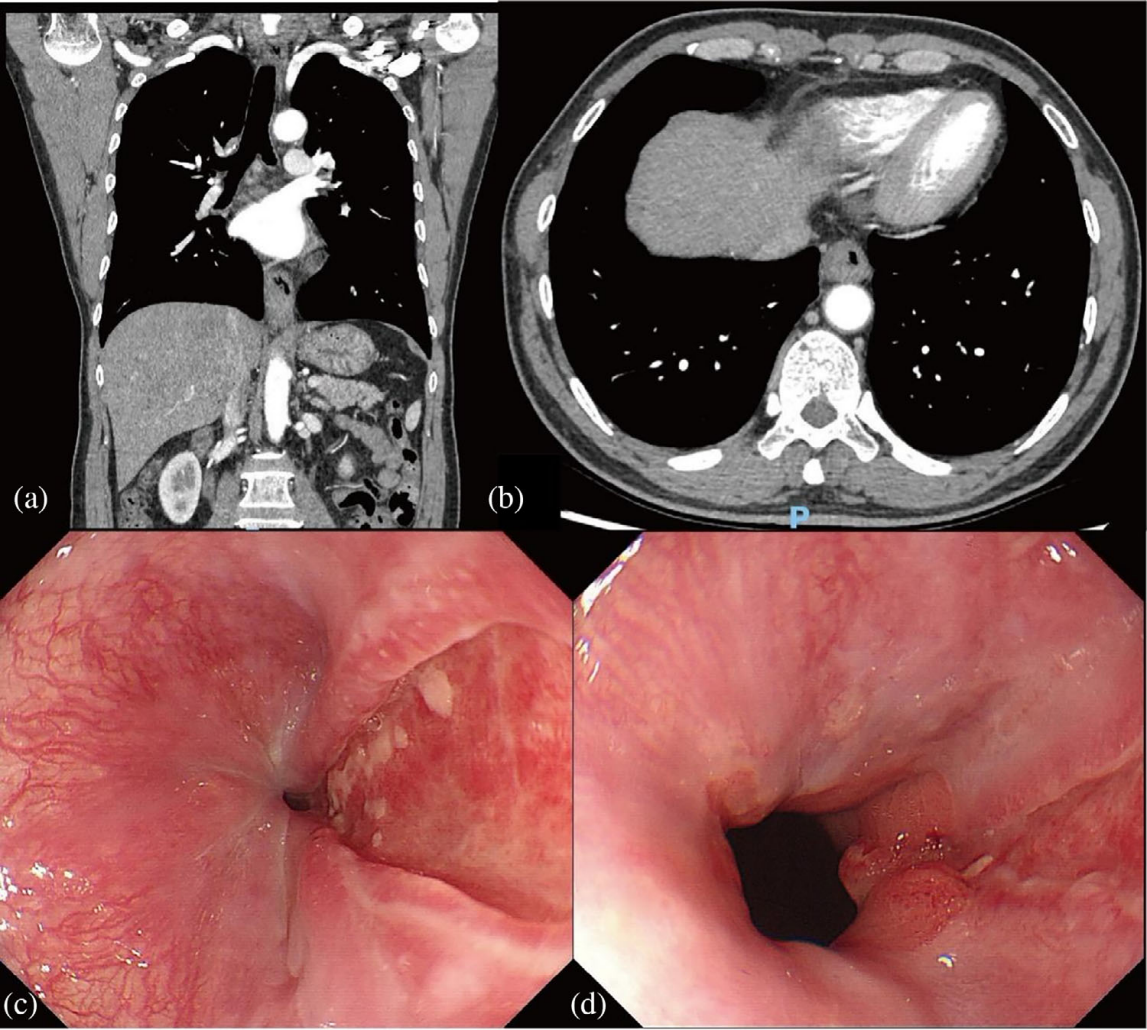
Reexamination of esophageal hematoma after treatment. (a, b) A computed tomography scan showed the wall of the esophagus gastric fundus and cardia were thickened. (c, d) The second esophagogastroduodenoscopy showed longitudinal ulcers.
